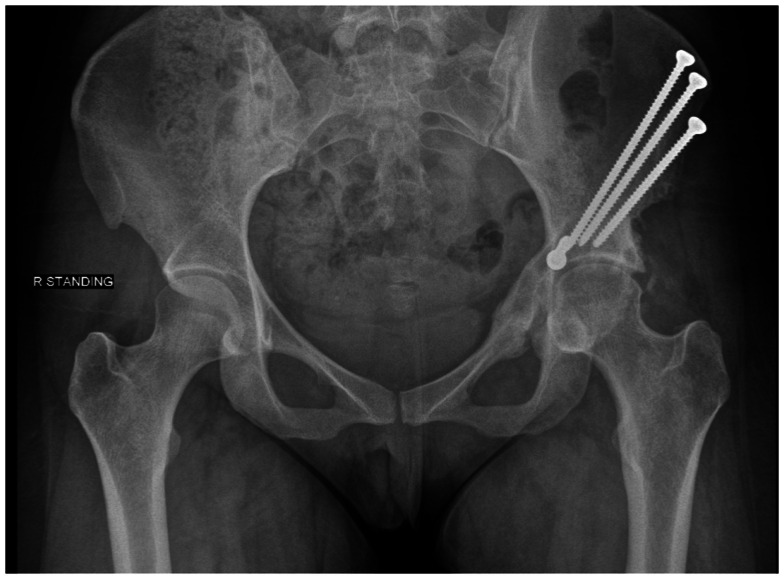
A postoperative radiograph of a periacetabular osteotomy secured with screws.
